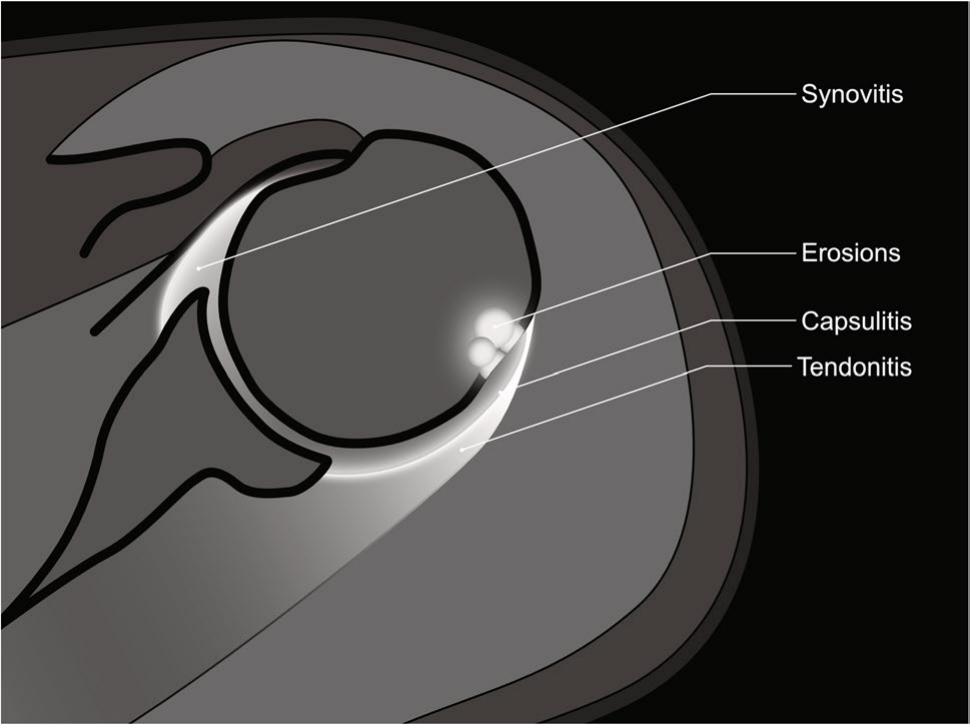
Typical, findings in chronic SIRVA on iv contrast-enhanced T1-weighted fat-saturated MRI
